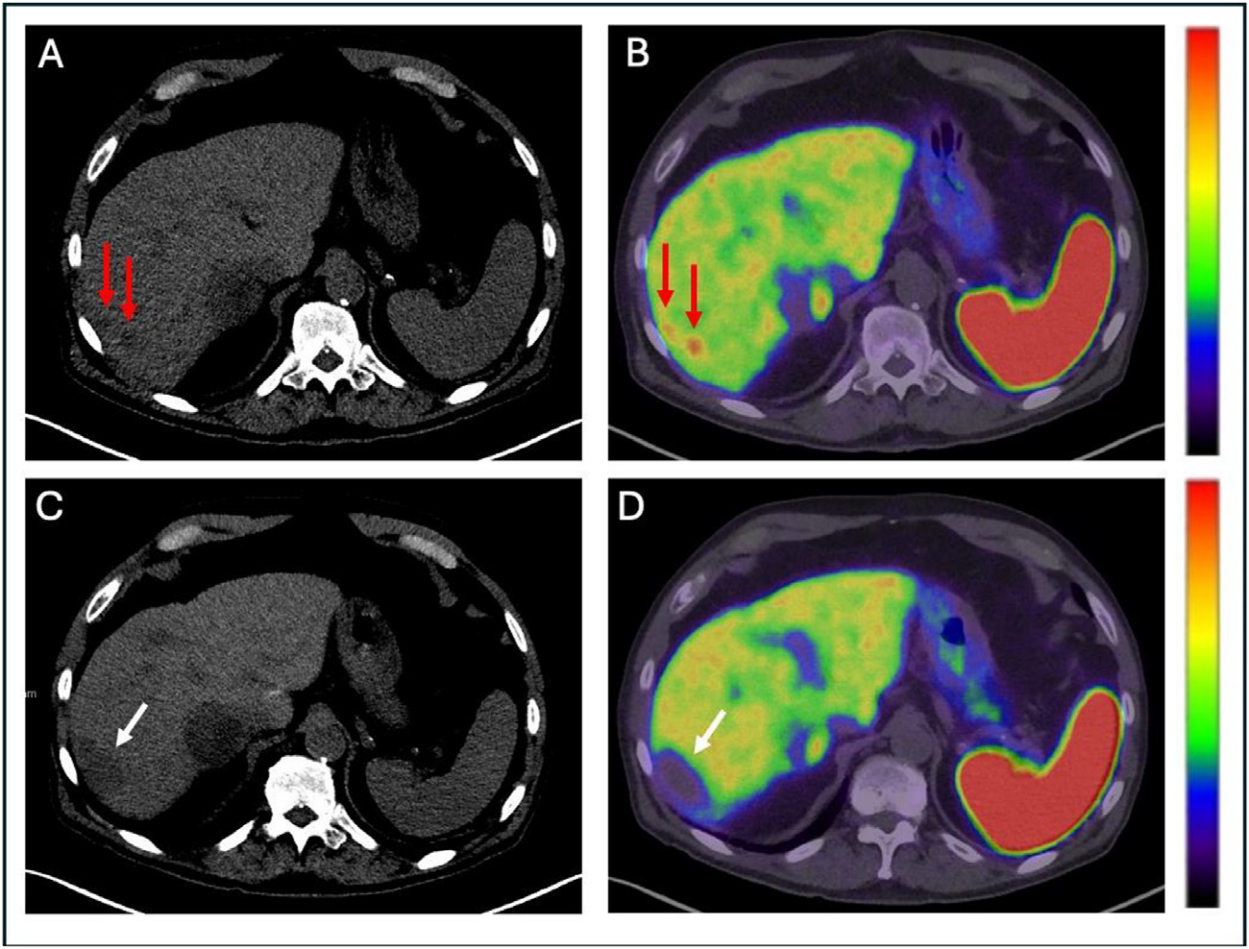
Axial abdominal CT (A) and corresponding fused DOTATATE PET-CT (windowed 0-15 SUV) (B) demonstrate 2 DOTATATE avid segment 6 lesions (red arrows) suspicious for recurrent metastatic meningioma. Two separate Neuwave PR15 XT probes were used to treat the Segment 6 lesions. Hydrodissection with saline was performed to insulate the abdominal wall. The settings for each ablation were 65 watts, for a total duration of 5 minutes. Axial abdominal CT (C) and fused DOTATATE PET-CT (D, windowed to 0-15 SUV) after thermal ablation demonstrate no DOTATATE avidity (white arrow), consistent with complete response. The combined ablation cavity was approximately 4 cm in diameter.
